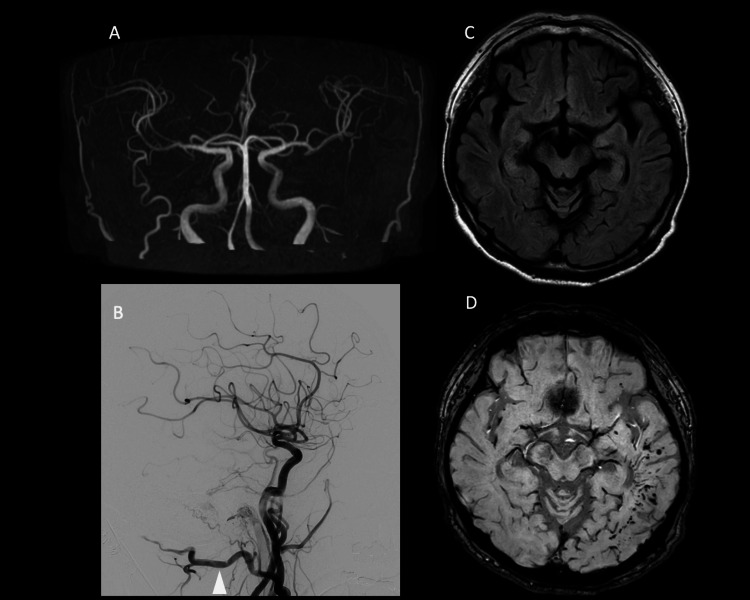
Post-treatment. MRA taken three months after treatment shows the disappearance of the dAVF (A). Cerebral angiography confirms the absence of the shunt (B: lateral view). SWI shows no significant change in venous dilation, but FLAIR imaging reveals a reduction in the hyperintense area (C, D) (occipital artery: white arrowhead). MRA: magnetic resonance angiography; dAVF: dural arteriovenous fistula; FLAIR: fluid-attenuated inversion recovery; SWI: susceptibility-weighted imaging
